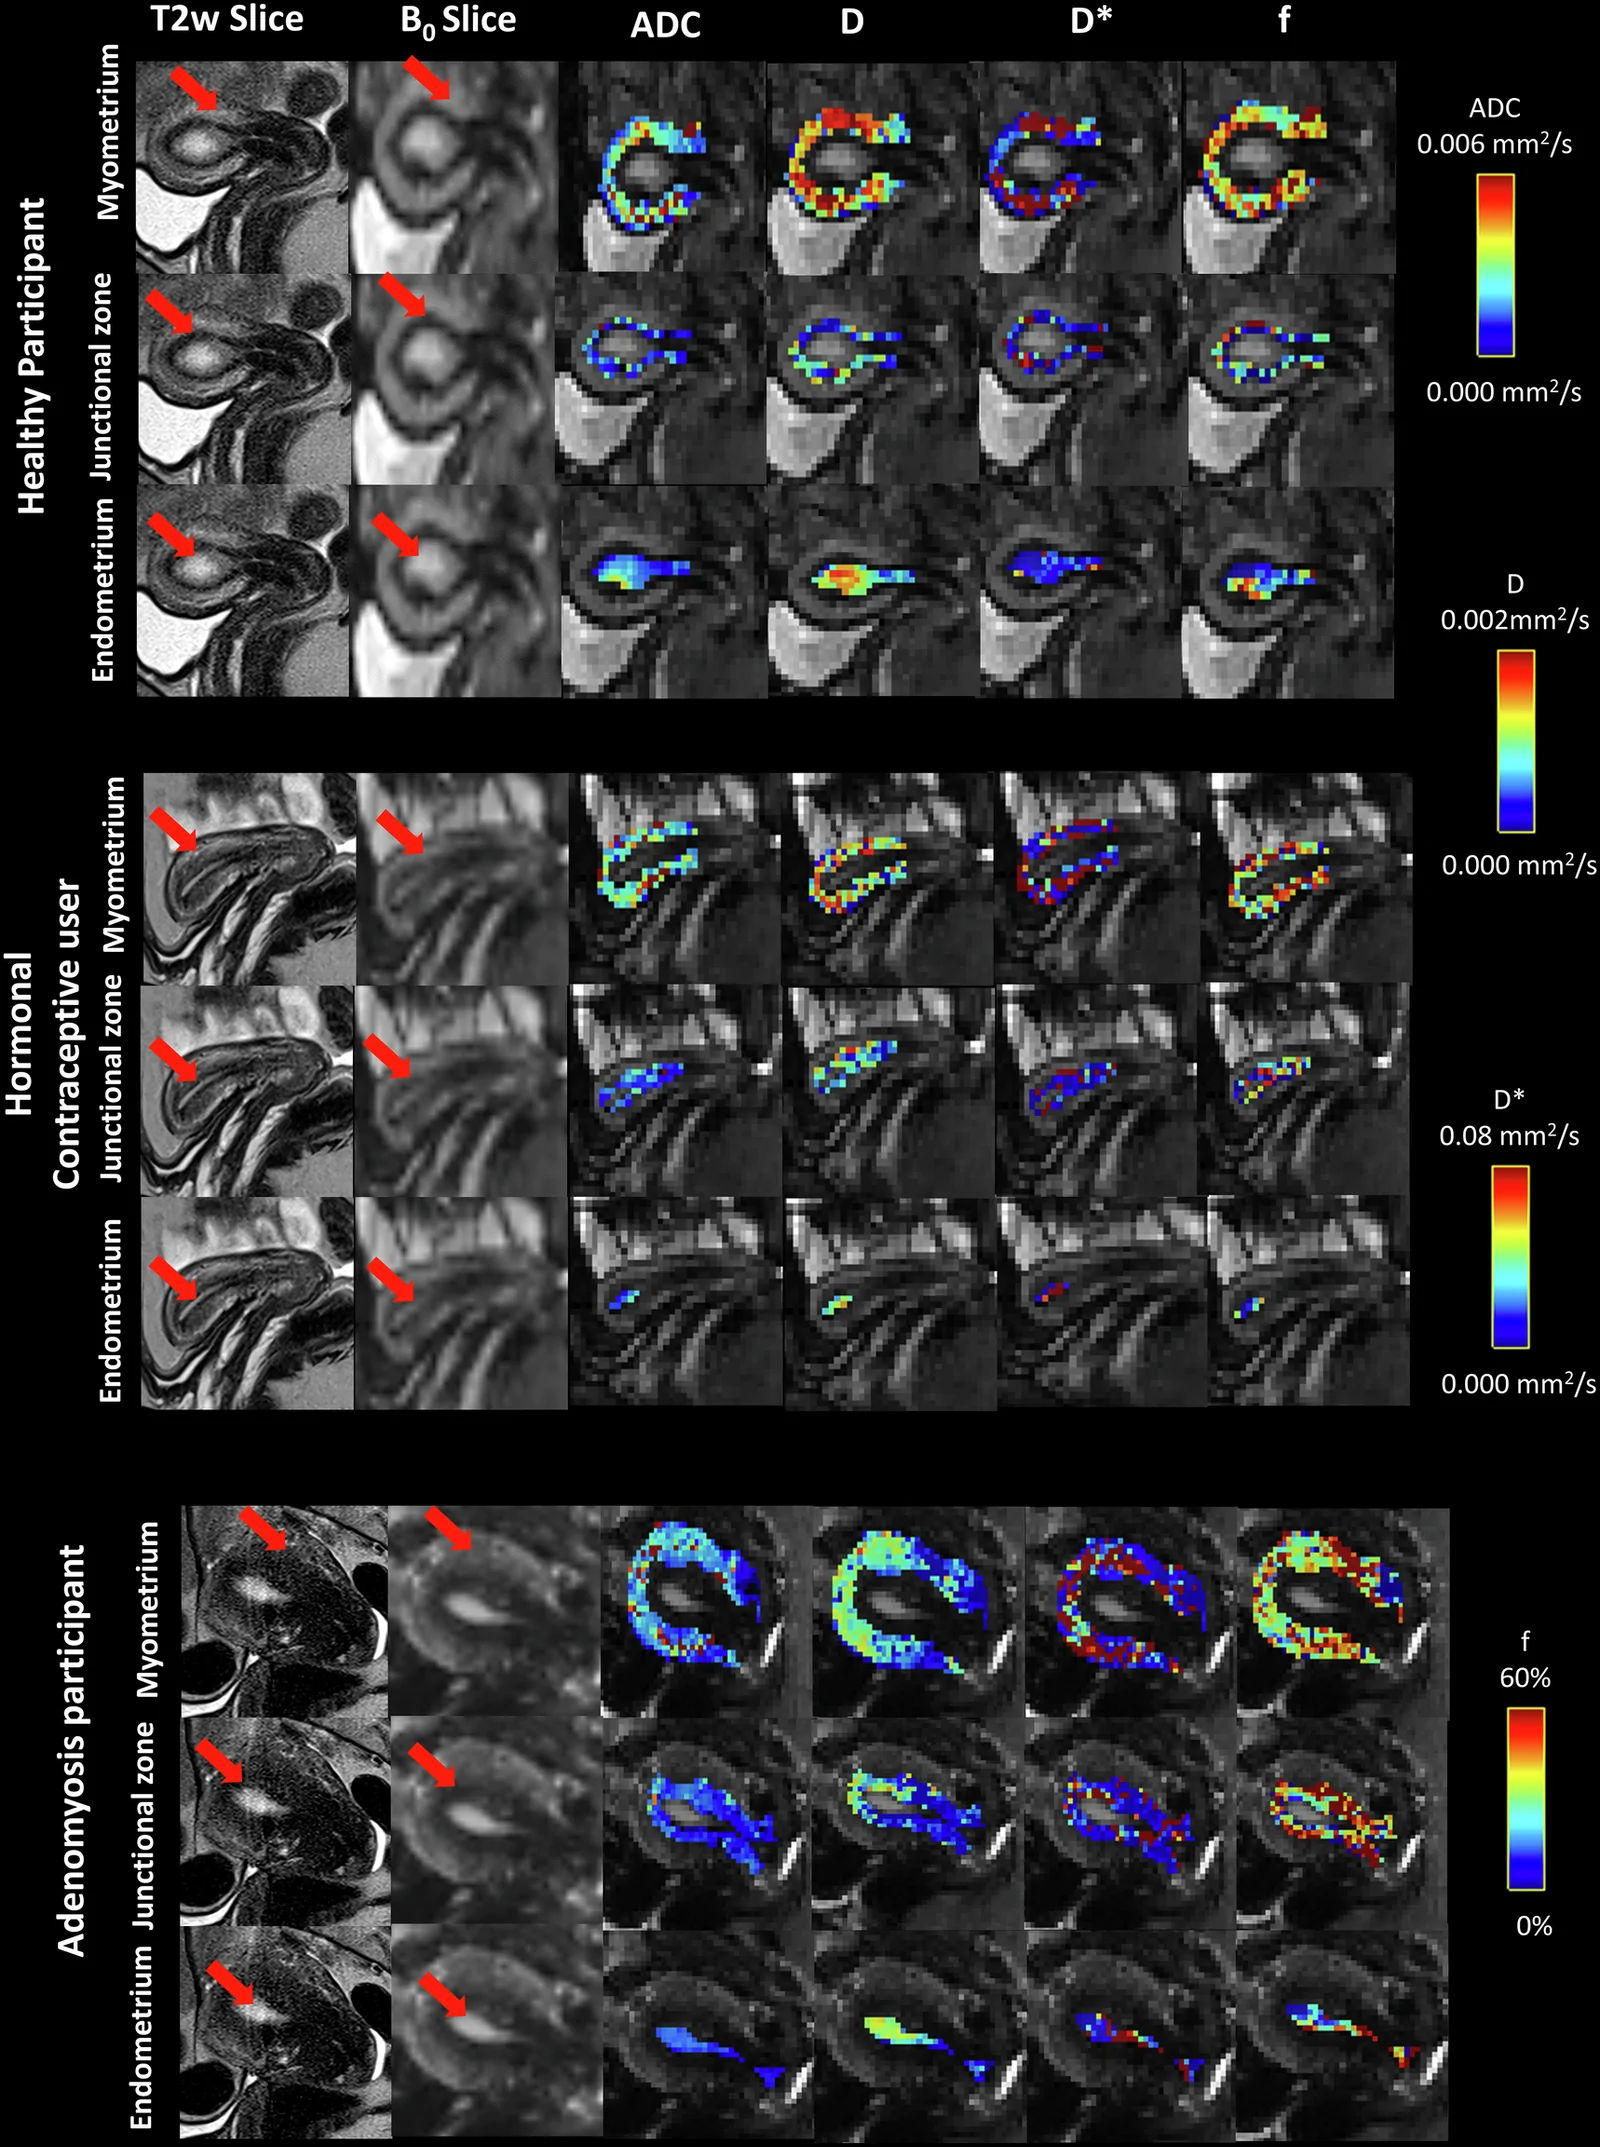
T2-weighted uterine mid-sagittal slice, apparent diffusion coefficient (ADC), diffusivity (D), pseudodiffusivity (D*) and perfusion fraction (f) maps of the three uterine layers, overlaid on the corresponding *b* = 0 s/mm² images, are shown for a healthy volunteer without and with contraceptive usage and a participant with adenomyosis. Shown in red arrows are representative uterine layers
